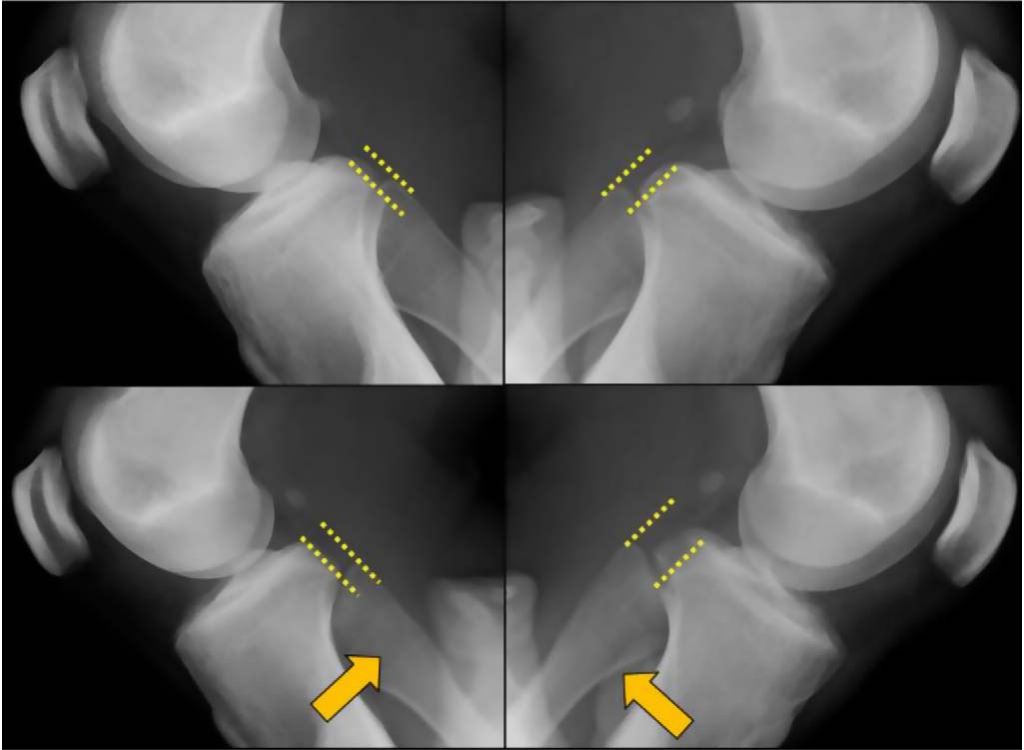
Anteroposterior stress X-ray imaging of the tibiofibular joint. Difference between the right and left sides are seen on anteroposterior stress X-ray images of the tibiofibular joint.
